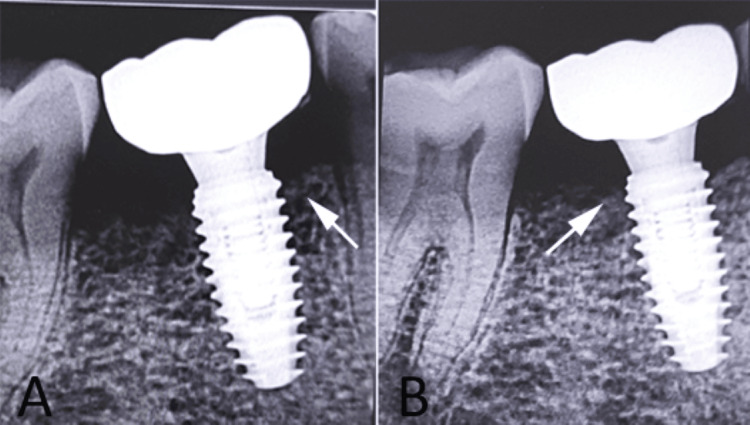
Follow-up evaluation findings A: post-loading radiograph showing stable crestal bone levels around the delayed implant (white arrow); B: three-year follow-up radiograph demonstrating maintained peri-implant bone levels (white arrow). Original patient images, used with the patient's permission.
